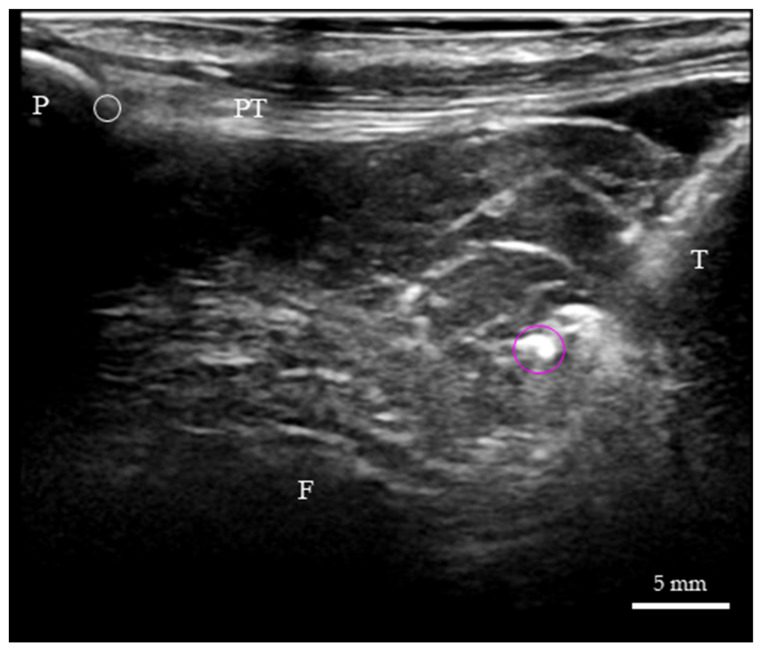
Long-axis ultrasound image of a Thiel-embalmed IPFP. A 14G needle is inserted into the AFC-SEG using an out-of-plane technique. white circle: anchor point; purple circle: 14G needle; P: patella; PT: patellar tendon; T: tibia; F: femur.
